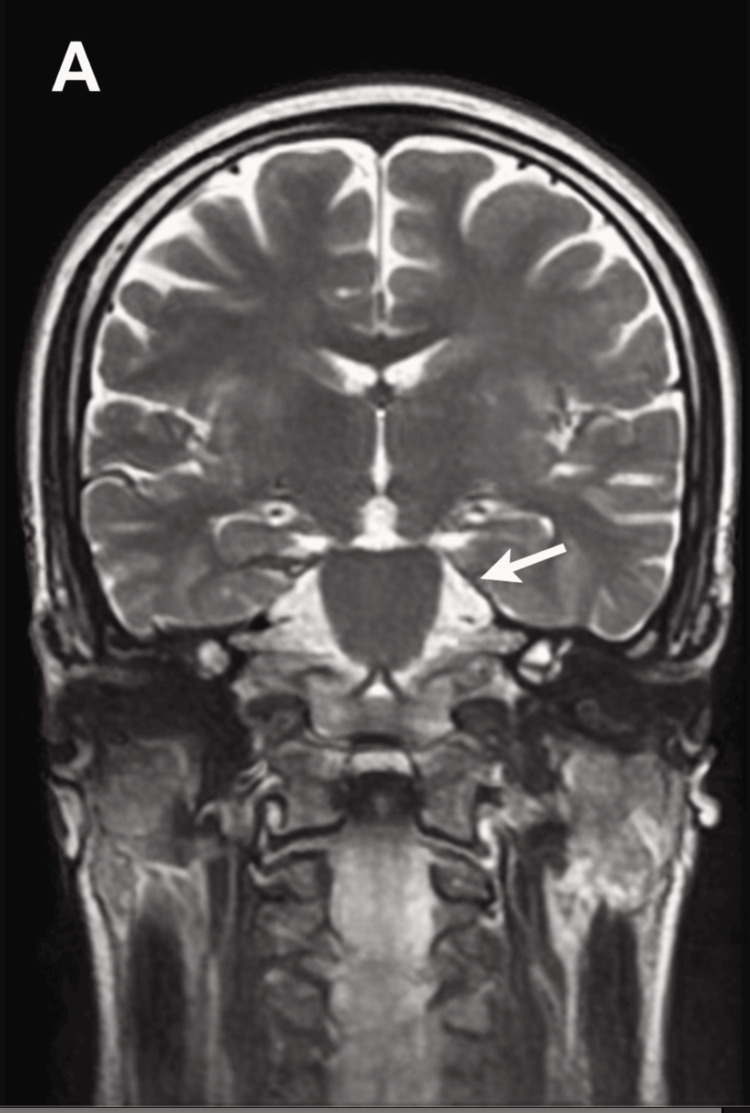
Brain magnetic resonance imaging (MRI). Axial T2-weighted brain MRI demonstrating no acute intracranial abnormalities. The arrow indicates a representative region of normal brain parenchyma.
